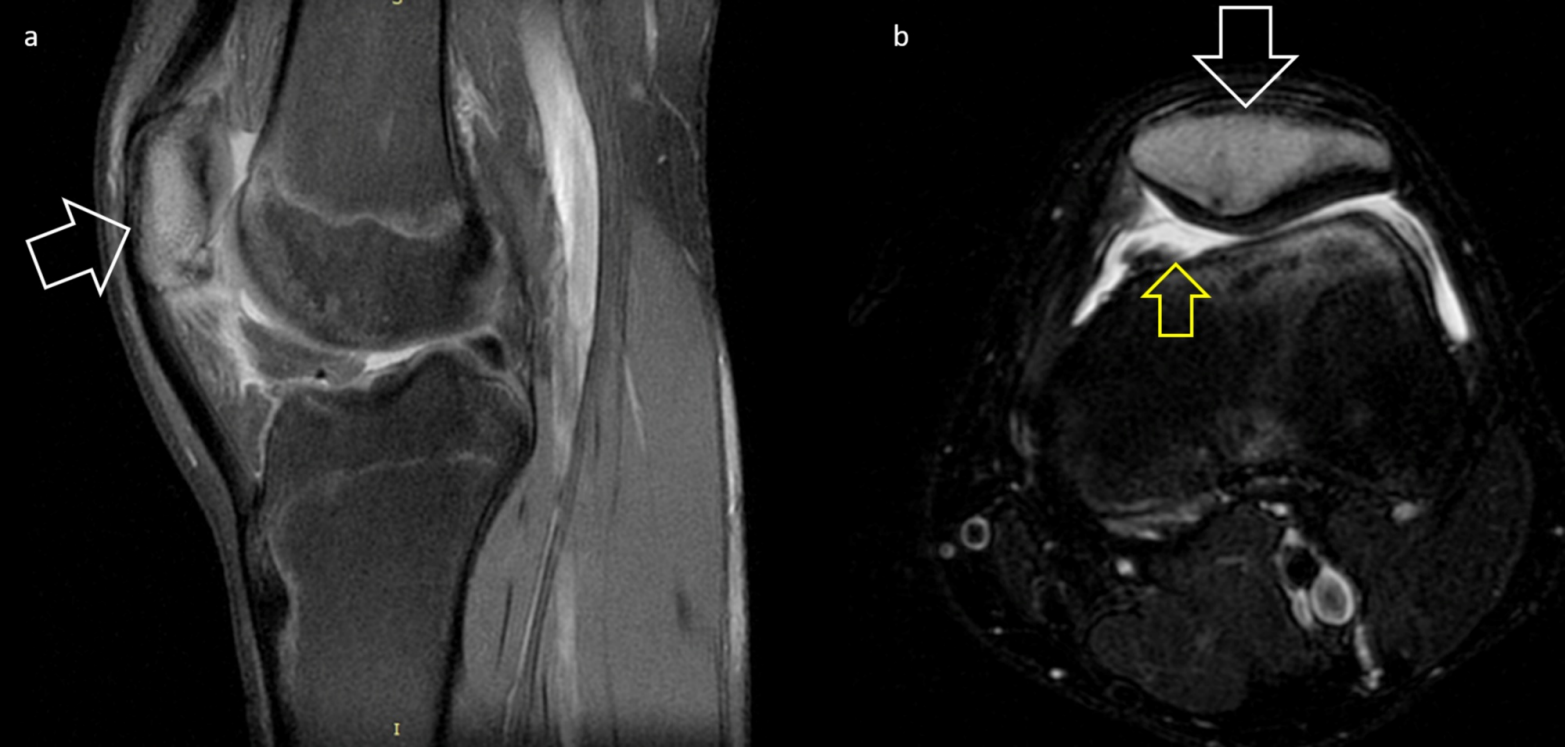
Third MRI scan, showing only non-specific findings, increased signal on T2 sequence, and mild effusion a. Sagittal view, b. Transverse view White arrows: increased signal on T2 sequences, Yellow arrow: knee effusion T2: T2-weighted image
